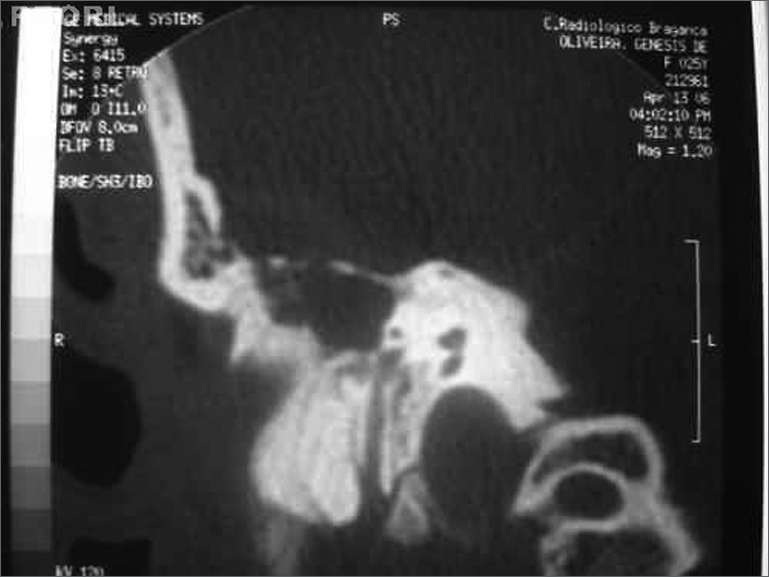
Computed tomography of the right ear (coronal section), showing erosion of the tegmen tympani.
